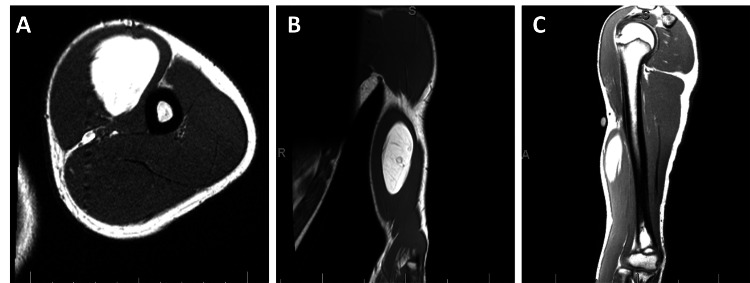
Transverse (A), coronal (B), and sagittal (C) plane T1-weighted MRI of the patient's upper extremity prior to excision.
